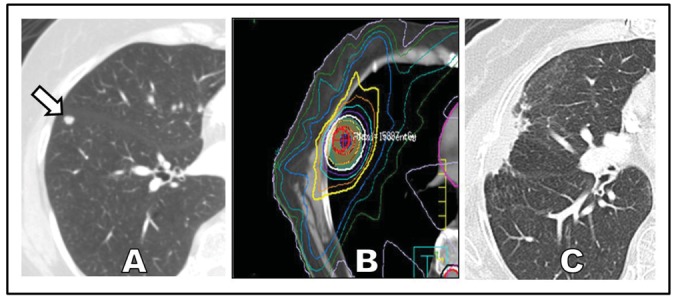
(A) Early-stage non-small cell lung cancer presenting as a peripheral nodule (white arrow) in a patient who was not a candidate for surgery; (B) Treatment with SABR to a dose of 50 Gy in 4 fractions (white isodose line); and (C) Surveillance computed tomography scan 3 years following treatment. The tumor has been replaced by focal consolidation.
